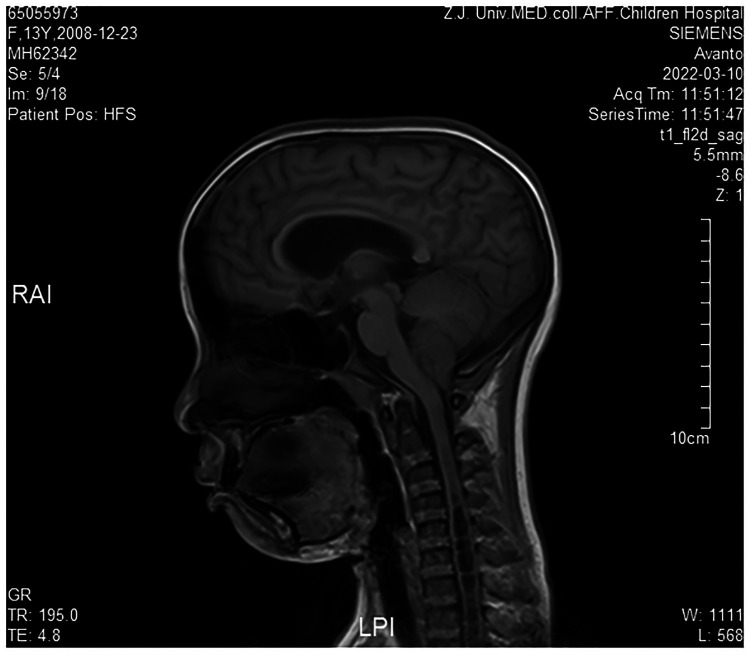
Brain MRI (transverse position).
